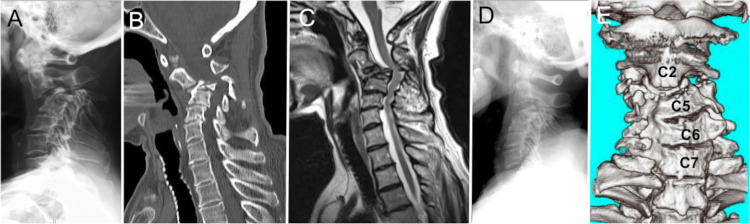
Radiological Images Upon Admission Kyphotic alterations due to the compression fracture of C3-C4 are evident. Tortuous modifications are noted in the spinal cord, although there is no apparent hyperintensity area within the spinal cord (A) X-ray image, (B) Sagittal CT image, and (C) Sagittal MR image. The kyphosis experiences slight correction with the application of a Halo-Vest (D). The midline cervical alignment continues to exhibit irregularity. (E) Three-dimensional reconstructed CT coronal image.
